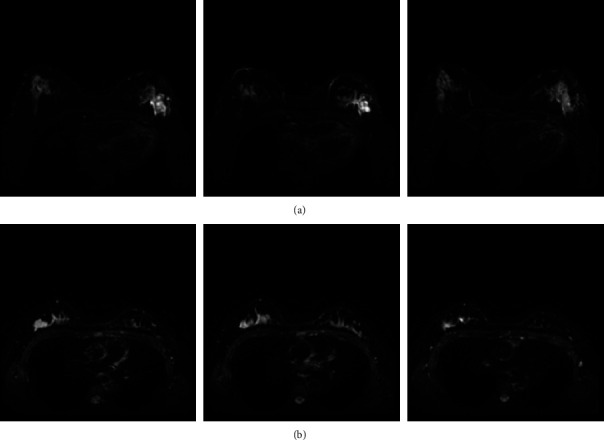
Partially scanned image based on (a) Ki-67 highly expressed DWI sequence and (b) 2. Ki-67 low-expression DWI sequence.
